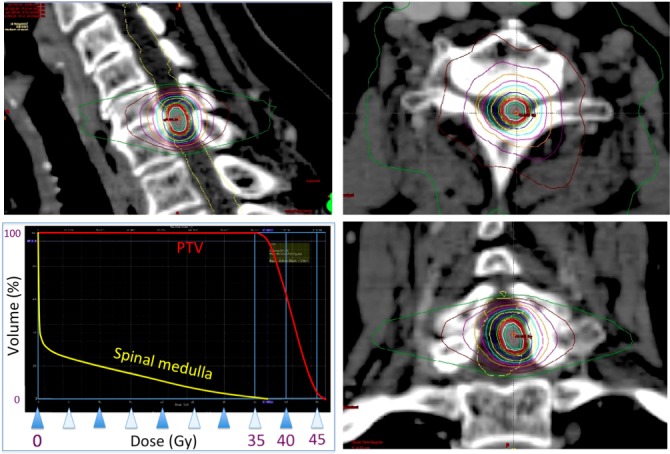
Pre-SRT CT of cervical lesion (Dose planning on Eclipse) Sagittal (*left upper*), axial (*right upper*), and coronal (*right lower*) images of iodine enhancement computed tomography (CT) on Eclipse (Varian, Tokyo) RTPS workstation. Dose-volume histogram is also shown (*left lower*). The enhanced intramedullary lesion was targeted with excellent conformity by VMAT simultaneously with sparing of the surrounding normal spinal medulla (*See also pre-SRT axial MRI of Figure 1 left*). A simultaneous boost up to 46.3 Gy was performed inside the tumor. 100% dose=39 Gy in 13 fractions, D95=95% dose.
